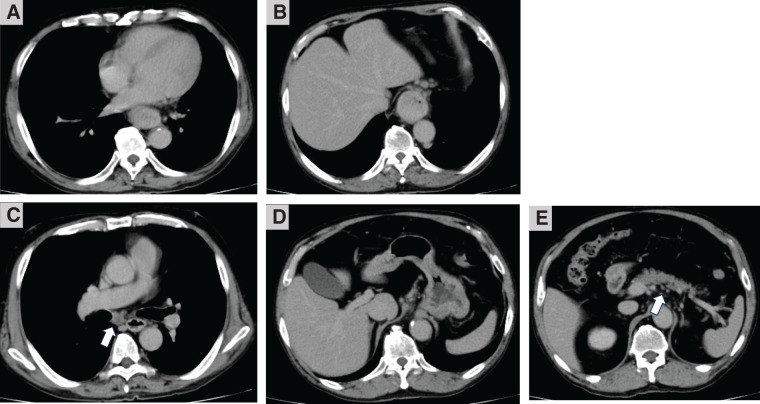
CT findings before esophagectomy. CT revealed wall thickening from the lower thoracic (A) to the abdominal esophagus (B), as well as enlarged bifurcational lymph nodes (C), lesser curvature of the stomach (D), and suprapancreatic lymph nodes (E). White arrows: enlarged lymph nodes.
